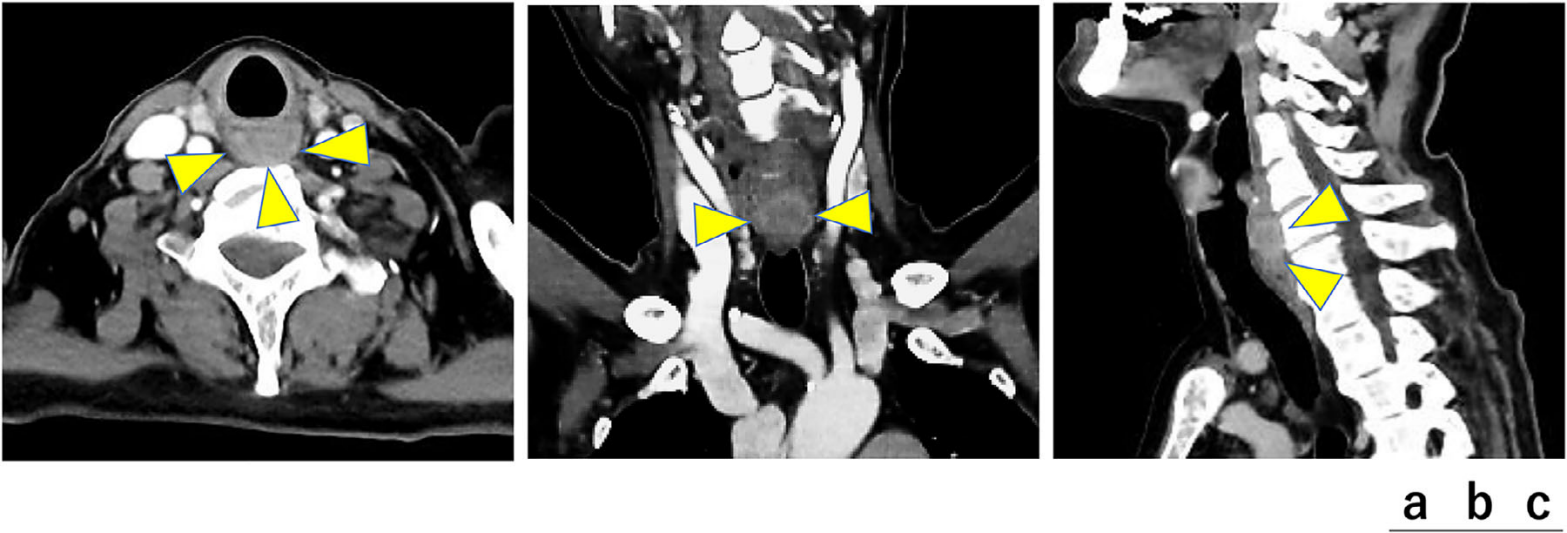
Contrast‐enhanced computed tomography (CT) of the head and neck. (a) Axial image. An approximately 20 mm mass with rim enhancement and relatively poor internal enhancement is seen posterior to the main bronchus (arrowhead). (b) Coronal image. A mass with rim enhancement and relatively poor internal enhancement is seen (arrowhead). (c) Sagittal image. At the level of the inferior border of the cricoid cartilage and ventral to the sixth cervical vertebra, a mass with rim enhancement and relatively poor internal enhancement is noted.
